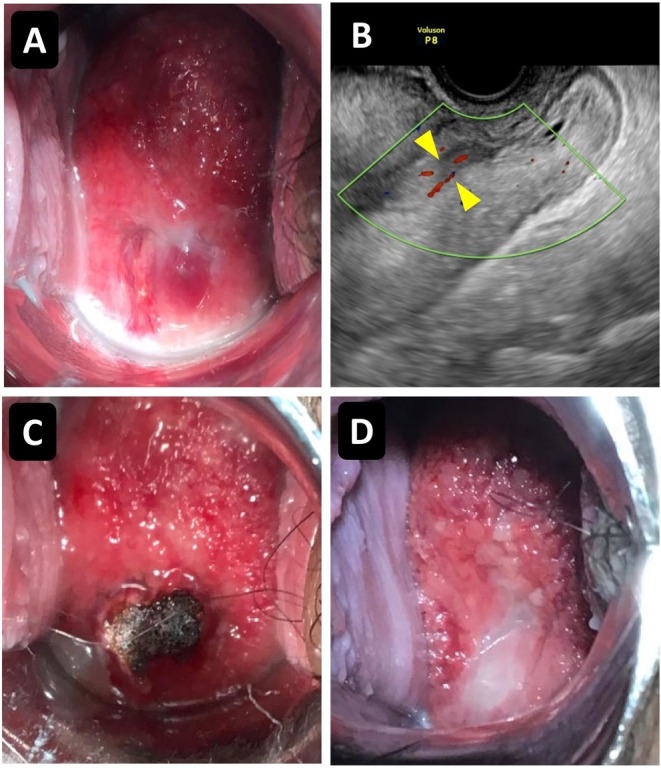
Case 3. (A) At 11 weeks and 3 days of gestation, two cervical polyps measuring 8 and 6 mm in diameter were observed, accompanied by bleeding from the polyps. (B) Color Doppler ultrasonography showed blood flow within the stalks of the cervical polyps located in the cervical canal (yellow arrows). (C) At 12 weeks and 4 days of gestation, cauterization was performed, consisting of three applications of 2–3 s each, resulting in successful hemostasis. After cauterization, a superficial eschar formed on the polyp surface, without thermal extension into the cervical canal. (D) At 13 weeks and 4 days of gestation, the cervical polyps were no longer visible on macroscopic examination.
